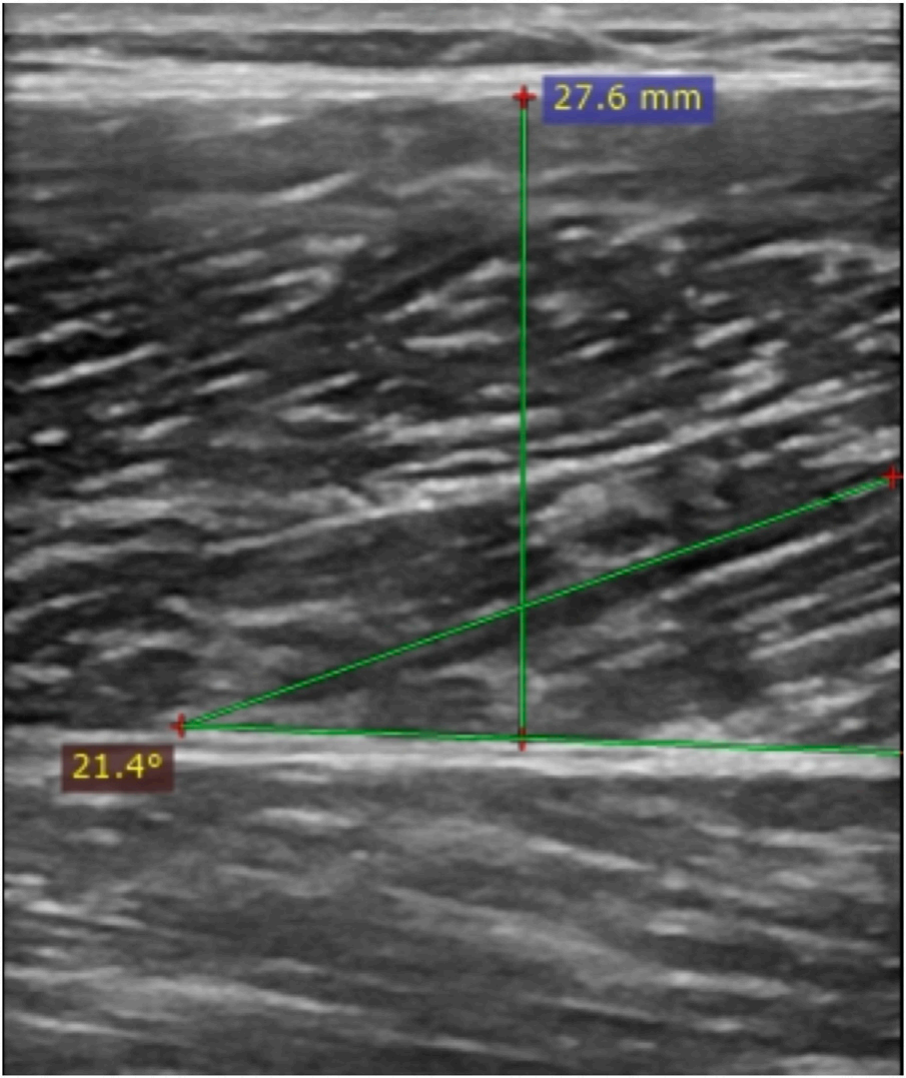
A typical musculoskeletal ultrasound image, demonstrating the thickness (MT) and pennation angle (PA) of the vertical muscle layers between the superficial fascia (SA) and deep fascia (DA) (indicated by the green lines).
